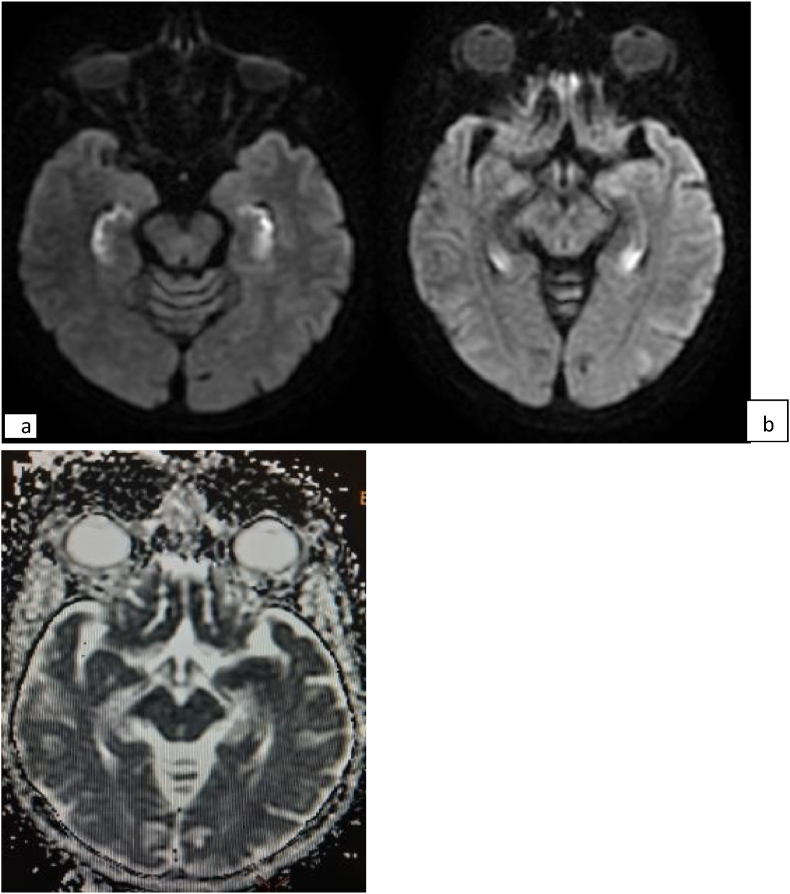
DWI shows diffuse bilateral hippocampal diffusion hyper intensity (a), without diffusion restriction on ADC map (b).
